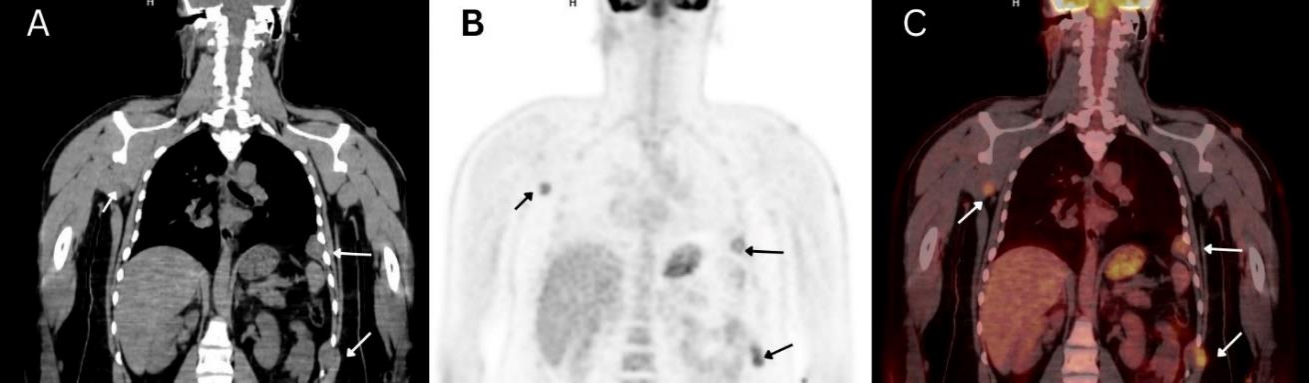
Coronal CT (A), PET (B), and fused images (C) demonstrate hypermetabolic two left pleural and intercostal soft tissue masses, as well as, a soft tissue lesion in the right axillary
